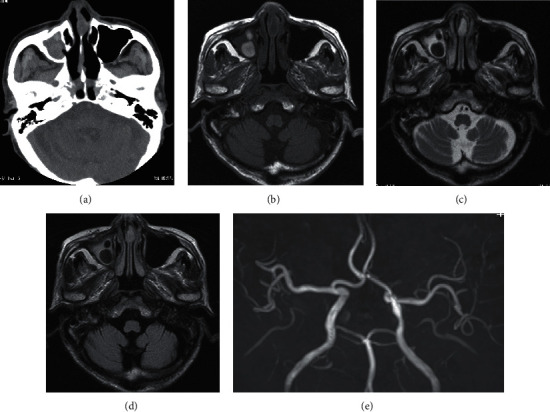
One month before admission: imaging included CT (a), T1-(b), and T2-weighted (c) images, fluid attenuated inversion recovery images (d), and MRA (e).
